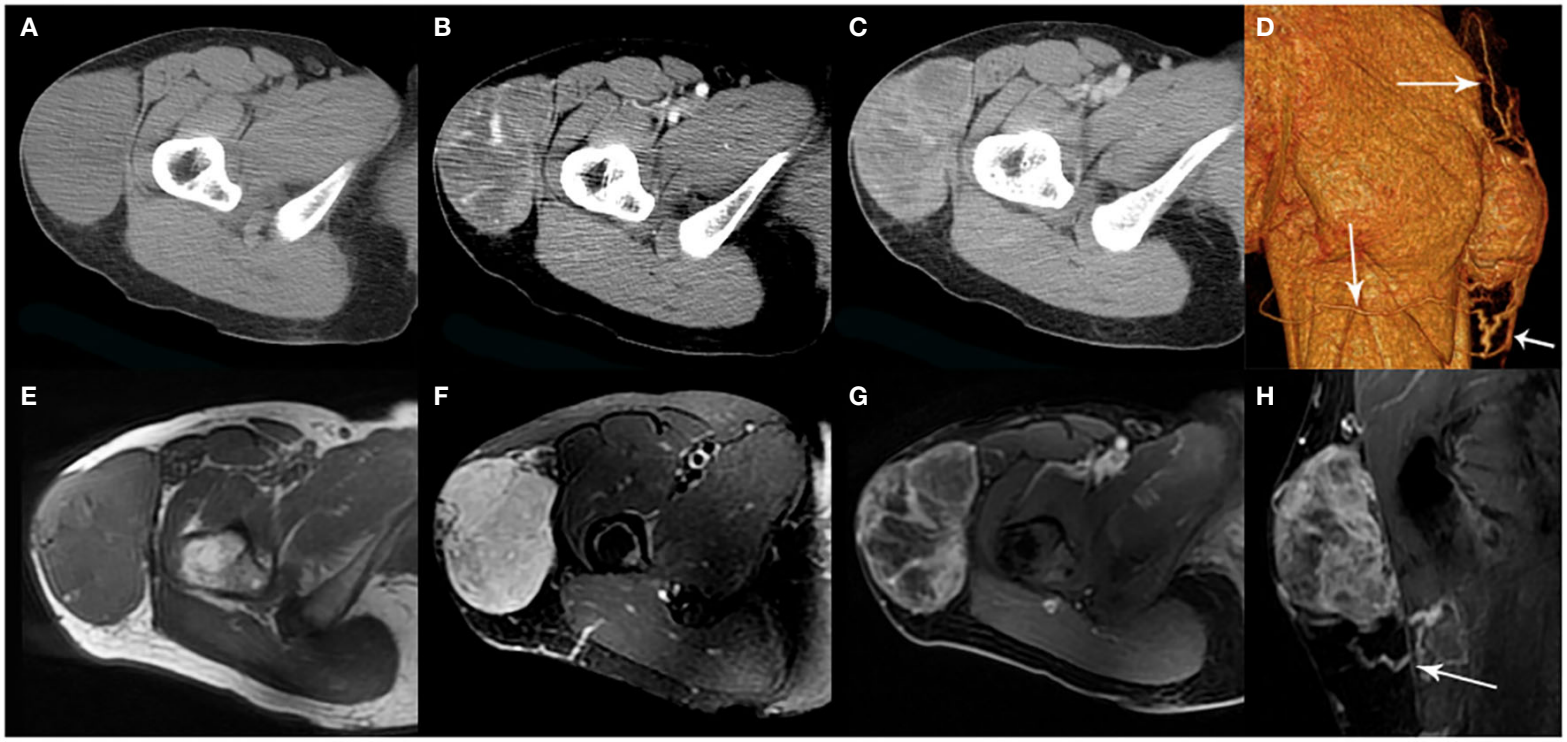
A 35-year-old woman with SFT. (A) Precontrast CT scan shows a mass in the proximal right thigh. (B) Multiple enlarged intratumoral vessels are seen in the arterial phase on post-contrast CT image. (C) Heterogeneous marked enhancement is detected in the venous phase. (D) Collateral feeding arteries are detected on 3D VR image (white arrows). (E) The tumor shows low intensity on T1-weighted image. (F) Areas of hypointensity and multiple flow voids were seen on T2-weighted image. (G, H) Heterogeneous moderate to marked enhancement and prominent collateral feeding artery (white arrow) are seen on postcontrast MR images.
